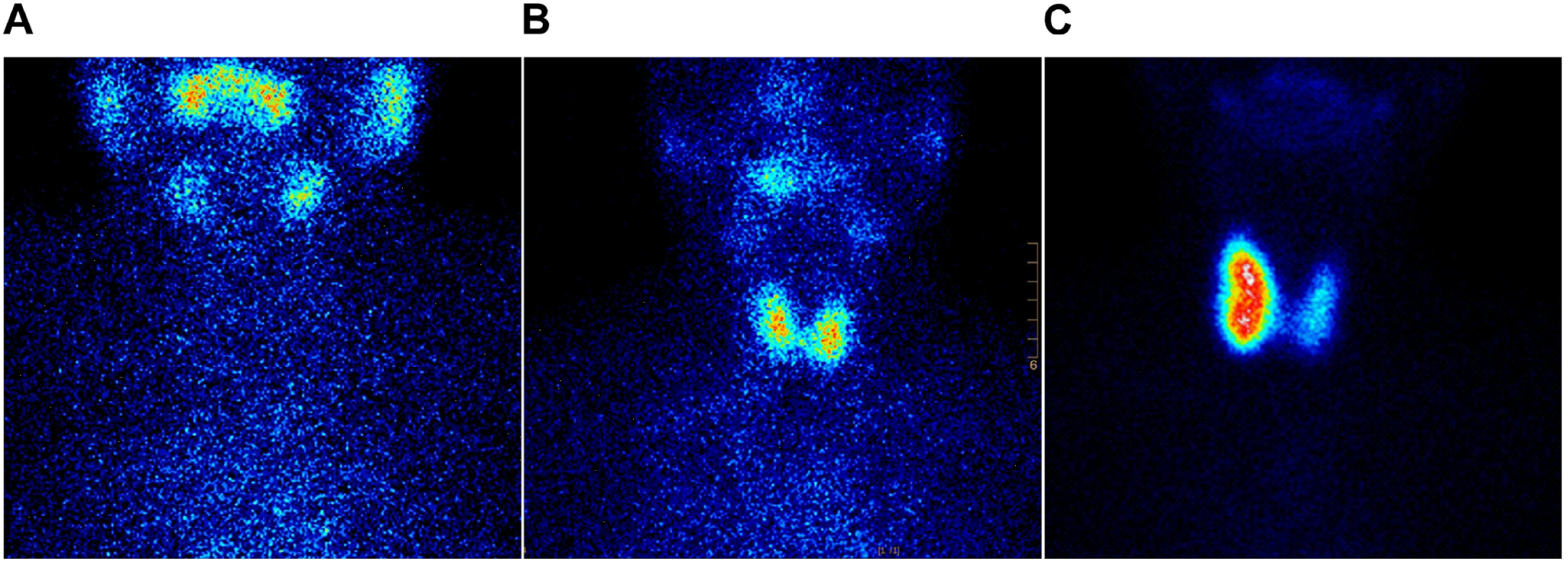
The figures of thyroid scintigraphy. We used ^99m^TcO_4_Na as radiotracer. The imaging equipment specifications including physical half‐life: 6.02 h, imaging time: 20 min, *Y*‐ray energy (keV): 140, and dose (MBq): 74–185. The figures of thyroid scintigraphy. (A) Cold nodule: the radiological distribution of nodules is sparse and defective; (B) warm nodules: the radioactive distribution of nodules is moderately average; (C) hot nodule: the radioactive distribution of nodules is concentrated.
